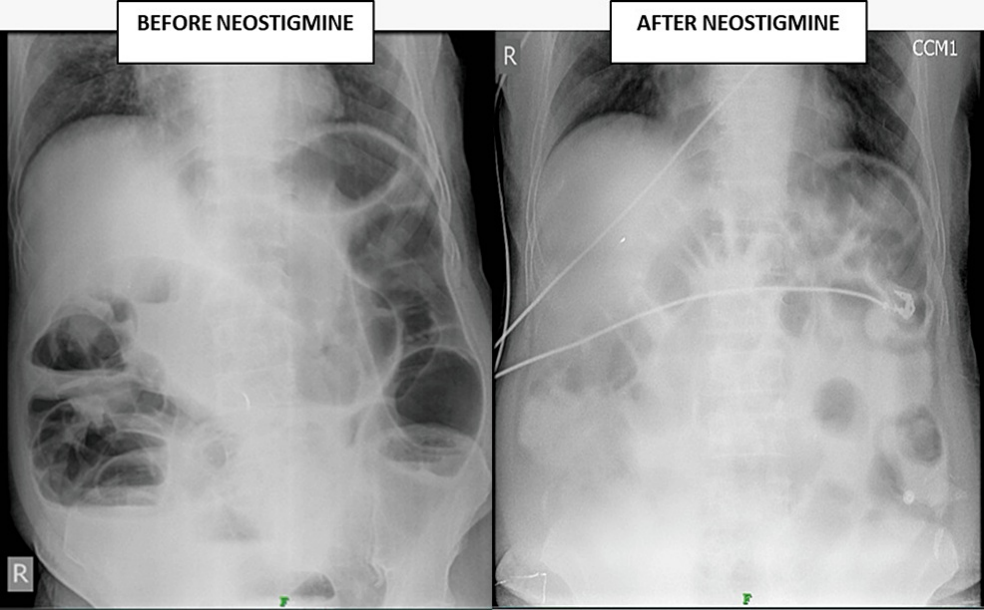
X-ray of the erect abdomen Demonstrates the decrease in air-fluid levels following the administration of injection neostigmine (2 mg IV).
